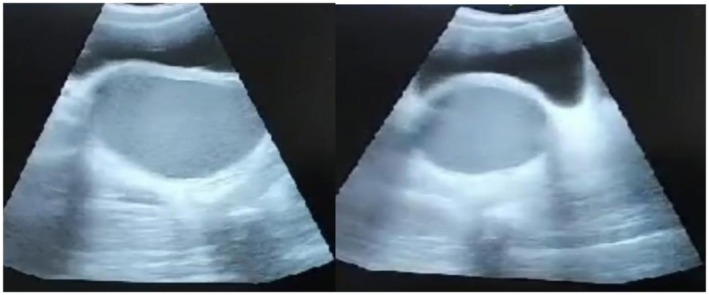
Presence of Hematocolpos (Ultrasound Scan).
